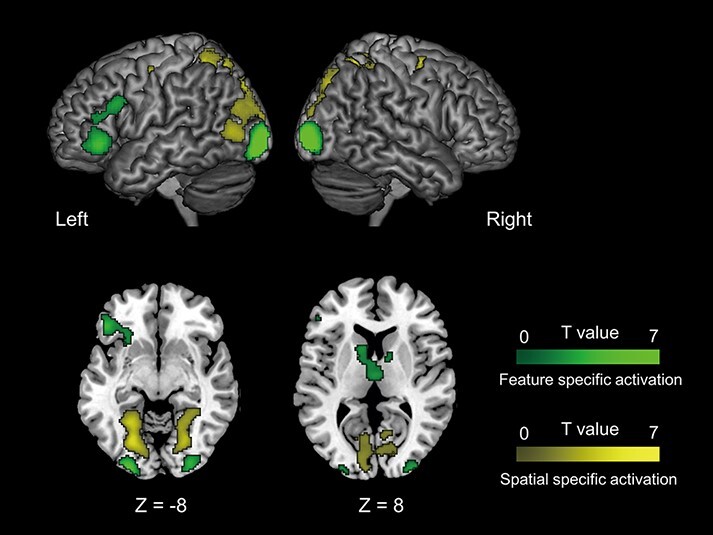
Task-related activation with object specificity. Feature-specific activation (green) and spatial location-specific activation (yellow) are superimposed on the template anatomical MRI scan.
